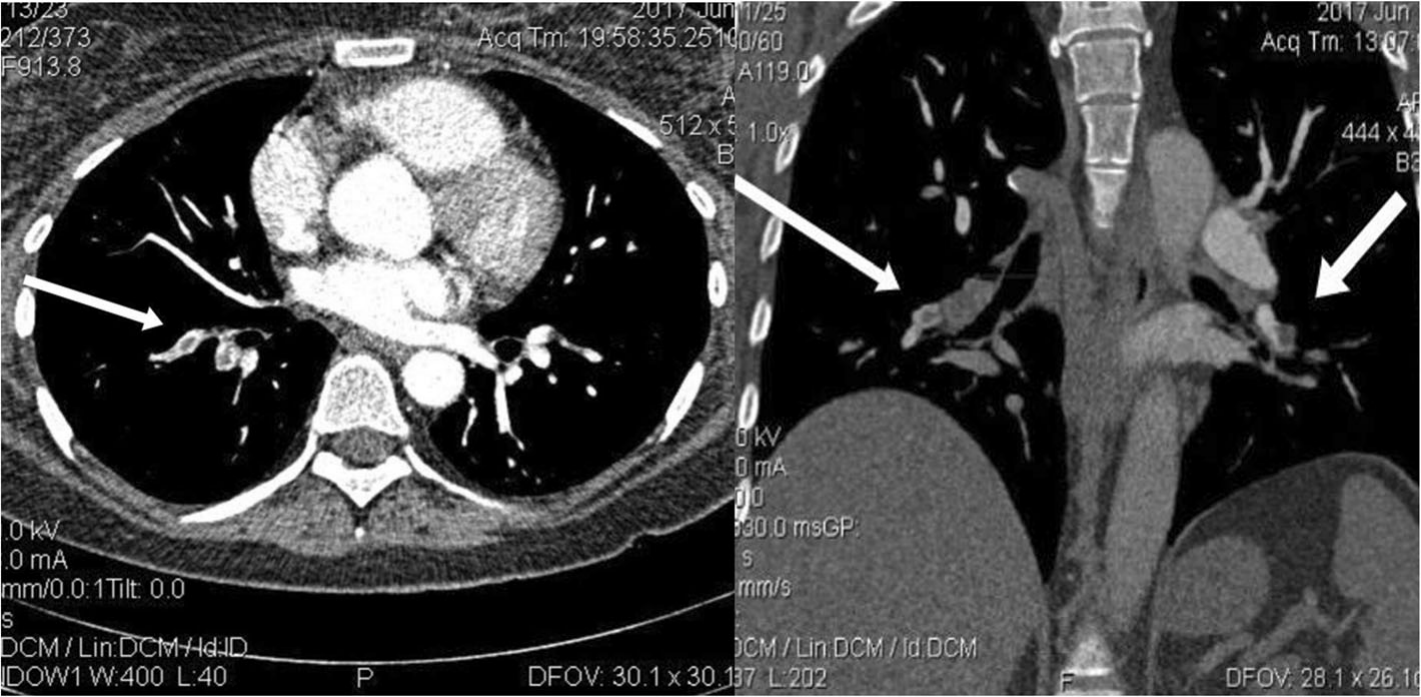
Computed tomographic angiography demonstrating filling defects in segmental right and left pulmonary arteries. The arrow is pointing to these filing defects
